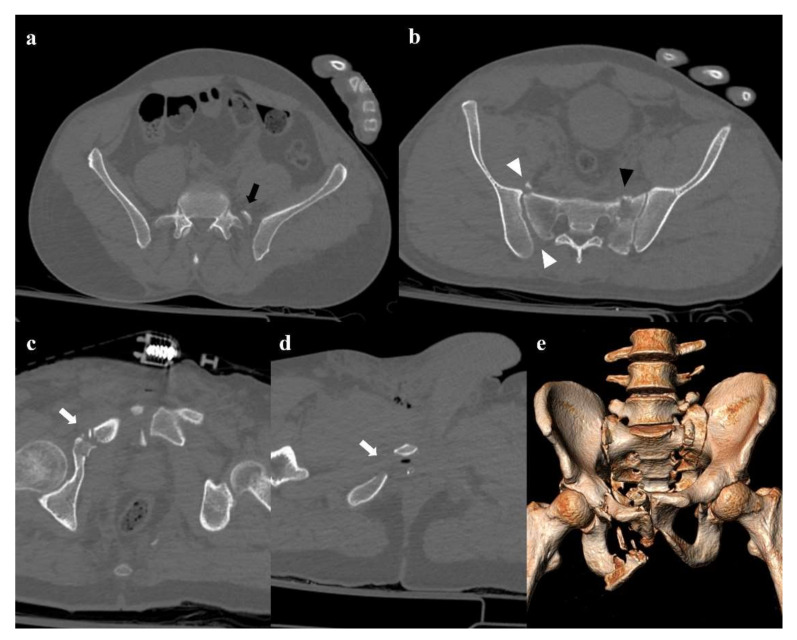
Vertical shear fracture. Axial CT images show avulsion of the left transverse process of the fifth lumbar vertebra (black arrow in (a)), fracture of the left side of the sacrum with involvement of the foraminal zone (black arrowhead in (b)), and fracture of the right superior and inferior pubic branches (white arrows in (c,d)). There is also a little fracture of the right wing of the sacrum, and a posterior widening of the right sacroiliac joint (white arrowheads in (b)). Three-dimensional volume-rendering CT reconstruction clearly depicts the instability of the pelvic ring, with the fracture of the left transverse process of the fifth lumbar vertebra and the cranial shift of the left hemipelvis (e).
